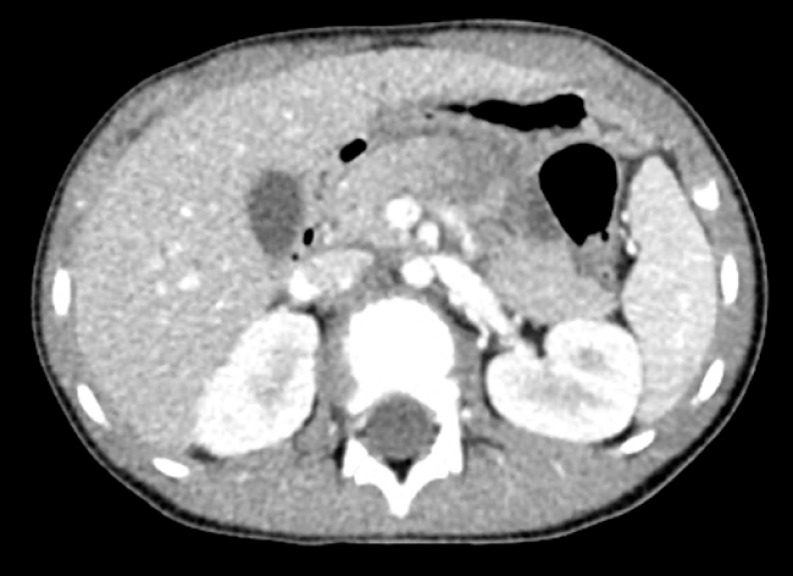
Computed tomography scan of abdomen (cross-sectional view) demonstrating pancreatic hematoma and edema suggestive of laceration of the pancreas.
